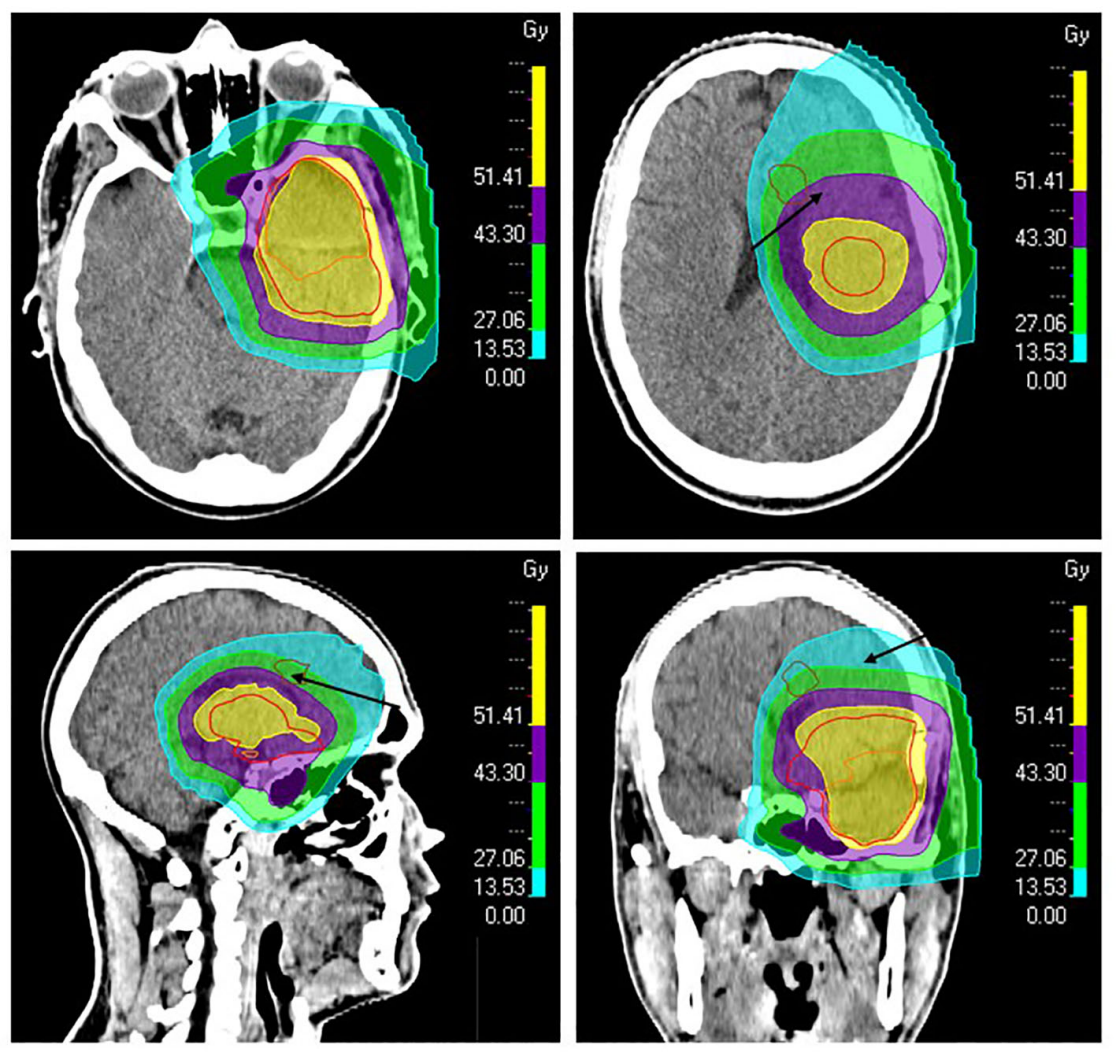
The patient’s proton plan showing isodose levels and radiotherapy target volumes. The resection cavity after primary surgery and the suspected residual tumor were delineated as gross target volume (GTV, orange). A margin of 15 mm was added to GTV to define the clinical target volume (CTV, red), which was also modified against natural anatomical barriers. The new lesion found by MRI three months after proton therapy was retrospectively delineated (brown, arrows) to calculate dose levels in the region of the lesion. The 51.4 Gray (Gy) (95%) isodose line is shown in yellow, 43.3 Gy (80%) in purple, 27.1 Gy (50%) in green, and 13.5 Gy (25%) in turquoise.
